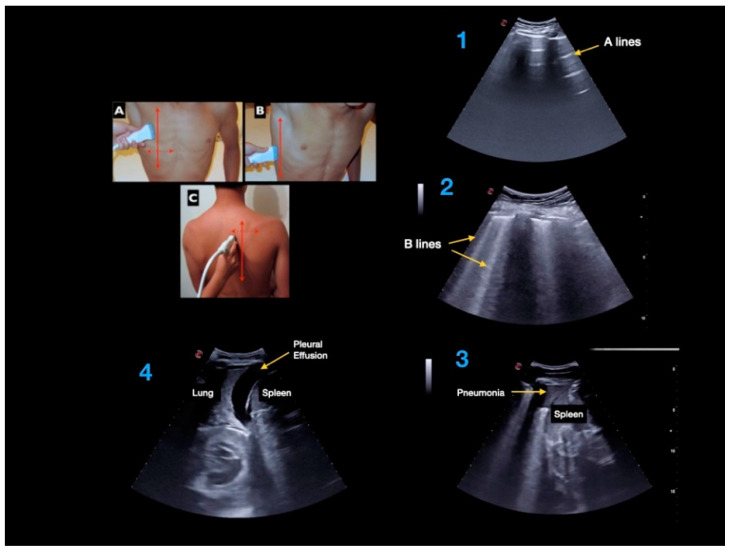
Lung ultrasound exam: (A) anterior, (B) lateral, and (C) posterior areas of both lungs. Lung ultrasound findings: (1) A-lines, (2) B-lines, (3) consolidation, and (4) pleural effusion.
